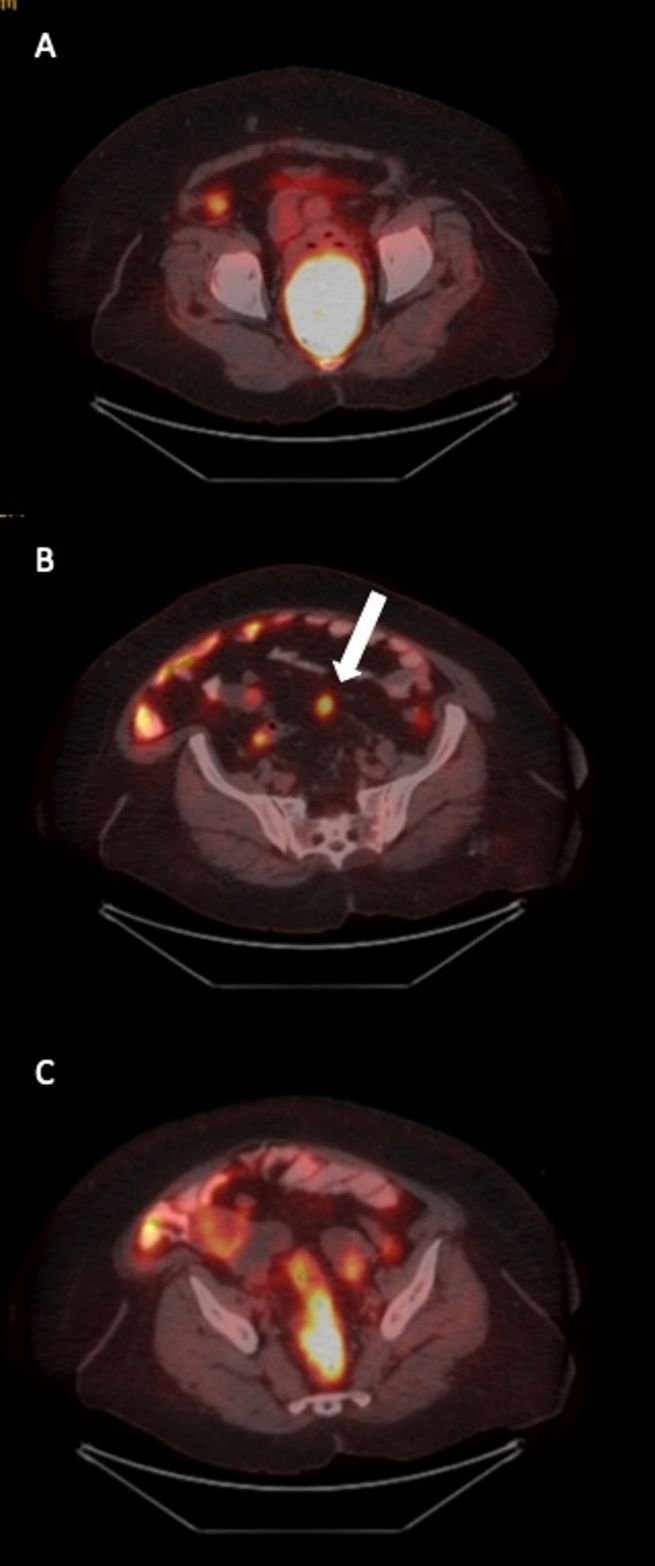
18 Fluoro-2-deoxyglucose positron emission tomography images. A: Axial image, increased FDG uptake in the mass measuring 9 x 7 x 9 cm in the cervix. B: Axial image, peritoneal implant. C: Axial image, increased FDG uptake in the ovarian masses. FDG: 18 Fluoro-2-deoxyglucose.
